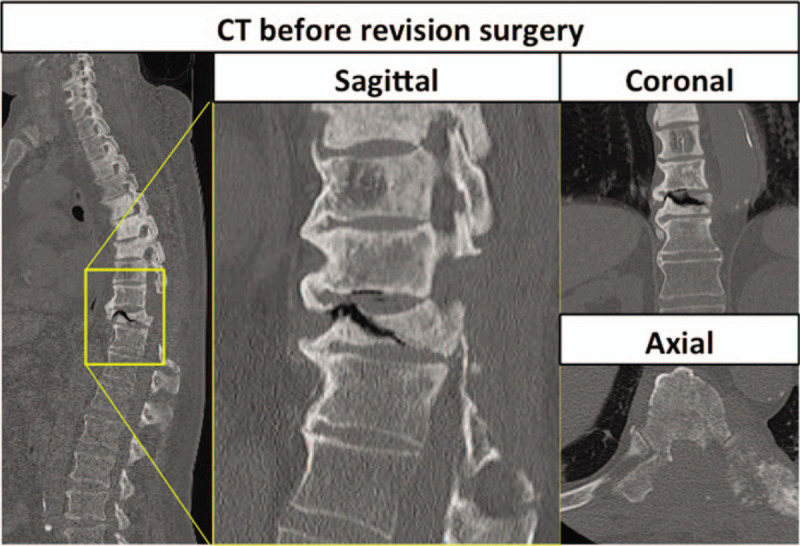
Preoperative computed tomography scan showing fracture of the vertebra at T10 with an accompanying intra-vertebral vacuum cleft sign.
